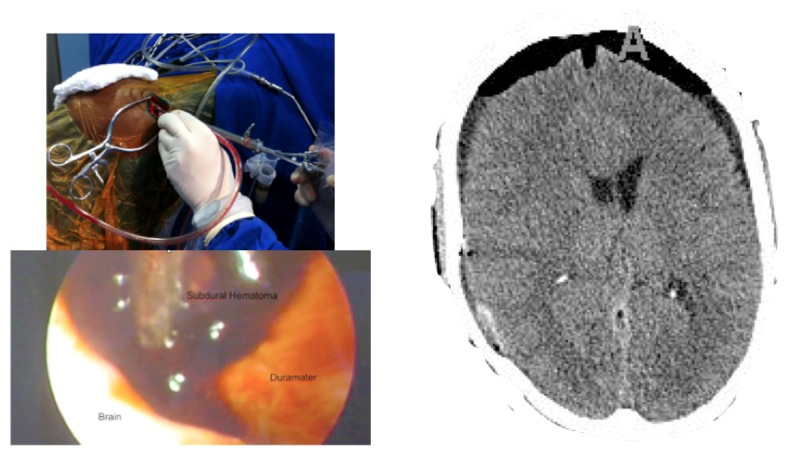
CASE 2. Post-operative results. During endoscopic resection is possible to insert an endoscope with aspirator or bipolar. On the left we see the positioning of the endoscope in one hand and aspirator on the other. It is also possible to see the intracranial intraoperative image. On the right, we see the postoperative CT scan with complete resection of the hematoma.
